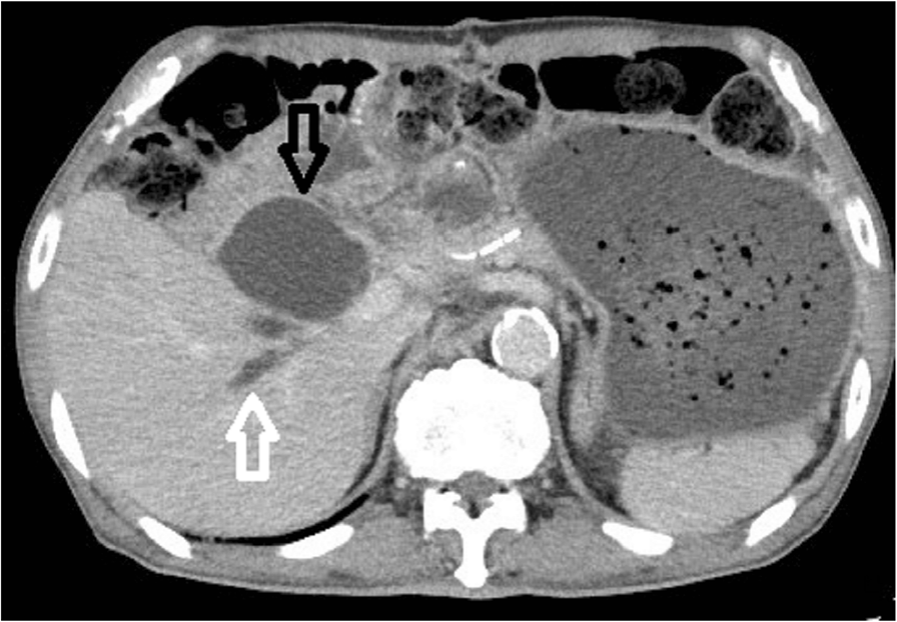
Postoperative computed tomography image: The *black arrow* indicates a tumor, suggestive of bile leakage, and the *white arrow* indicates a dilated posterior bile duct
